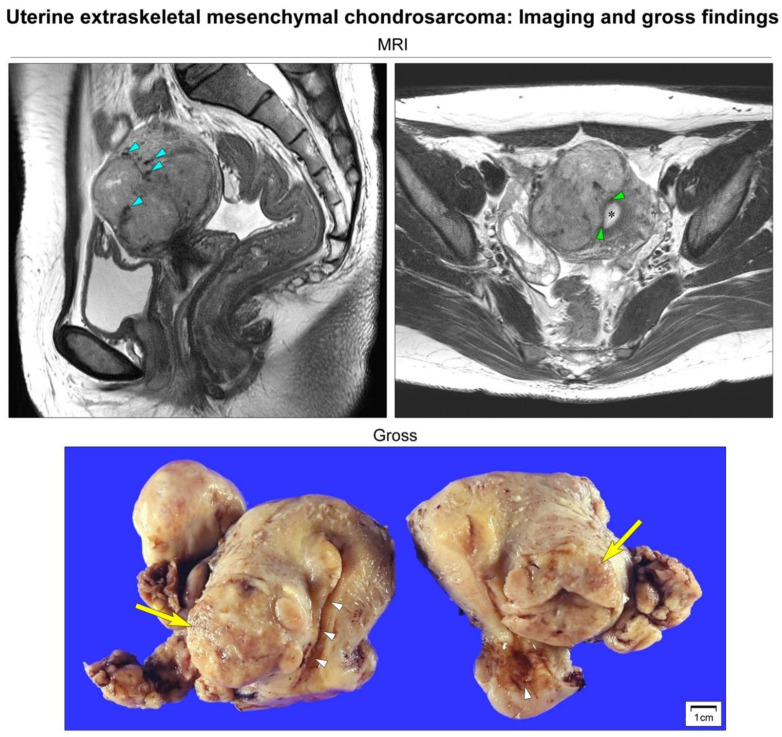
Imaging and gross findings. Abdominopelvic magnetic resonance imaging (MRI) reveals a well-circumscribed, multilobulated, solid mass measuring 6 cm located on the right side of the uterine corpus. T2-weighted sagittal imaging reveals an intramural uterine mass containing punctate, irregular-shaped, hypointense areas of calcification (blue arrowheads). T2-weighted axial imaging reveals that the mass is not connected to the endometrium (black asterisk). The endomyometrial junction (green arrowheads) is intact. Based on the preoperative impression of degenerated uterine leiomyoma or leiomyosarcoma, the patient underwent a total hysterectomy. Grossly, a lobulated, tan-white, rubbery mass (yellow arrows) appears to be confined within the right lateral wall of the uterus. The endocervical and endometrial mucosa (white arrowheads) are unremarkable.
